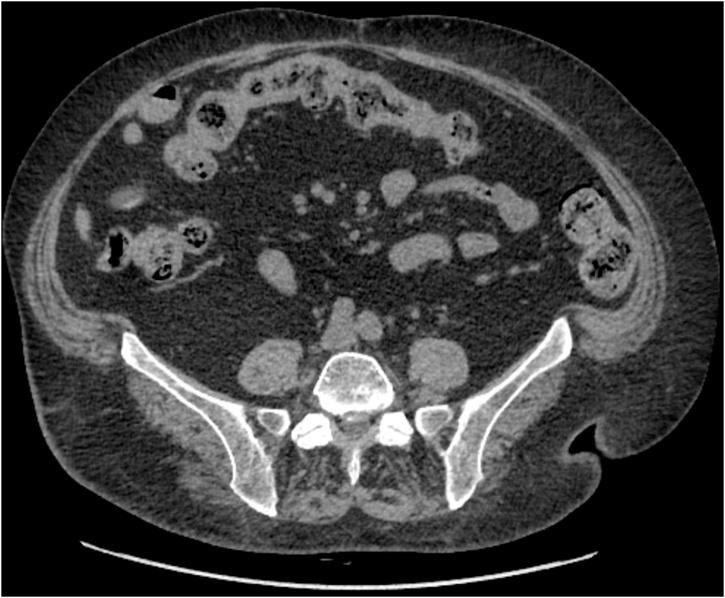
Abdominal CT scan after definitive cure of the incisional hernias.
